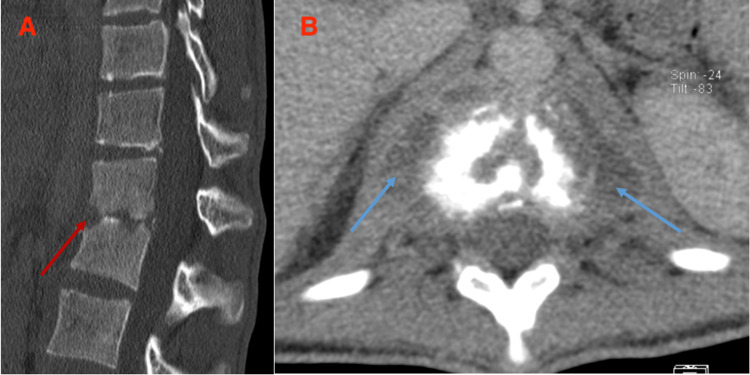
CT sagittal slice, bone window (Image A): destruction of the disc with erosion of the cortical bone (red arrow). CT axial slice, soft-tissue window (Image B): abscess collections (blue arrows).
